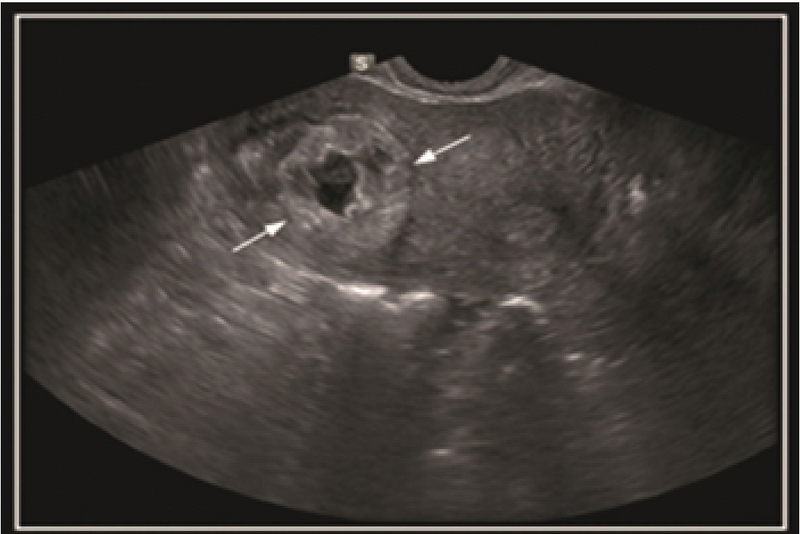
Interstitial pregnancy. Transverse transvaginal ultrasound image shows an eccentric gestational sac with an echogenic wall (arrows) partially surrounded by myometrium.
